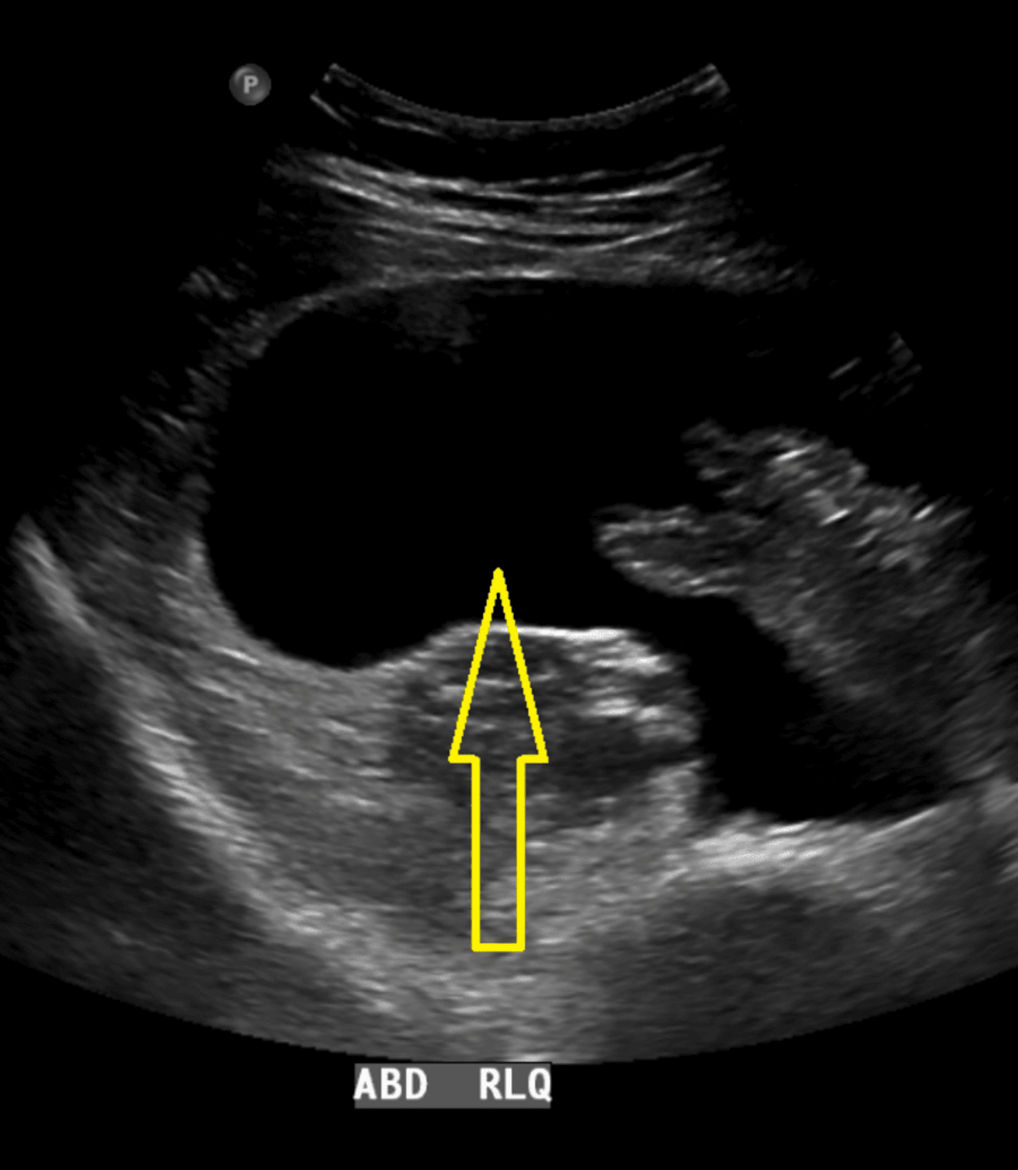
Ultrasound of the abdomen This ultrasound image of the right lower quadrant (RLQ) of the abdomen shows a large anechoic area (indicated by the yellow arrow), suggestive of a fluid-filled structure. The surrounding hyperechoic areas may represent the bowel and adjacent soft tissues.
